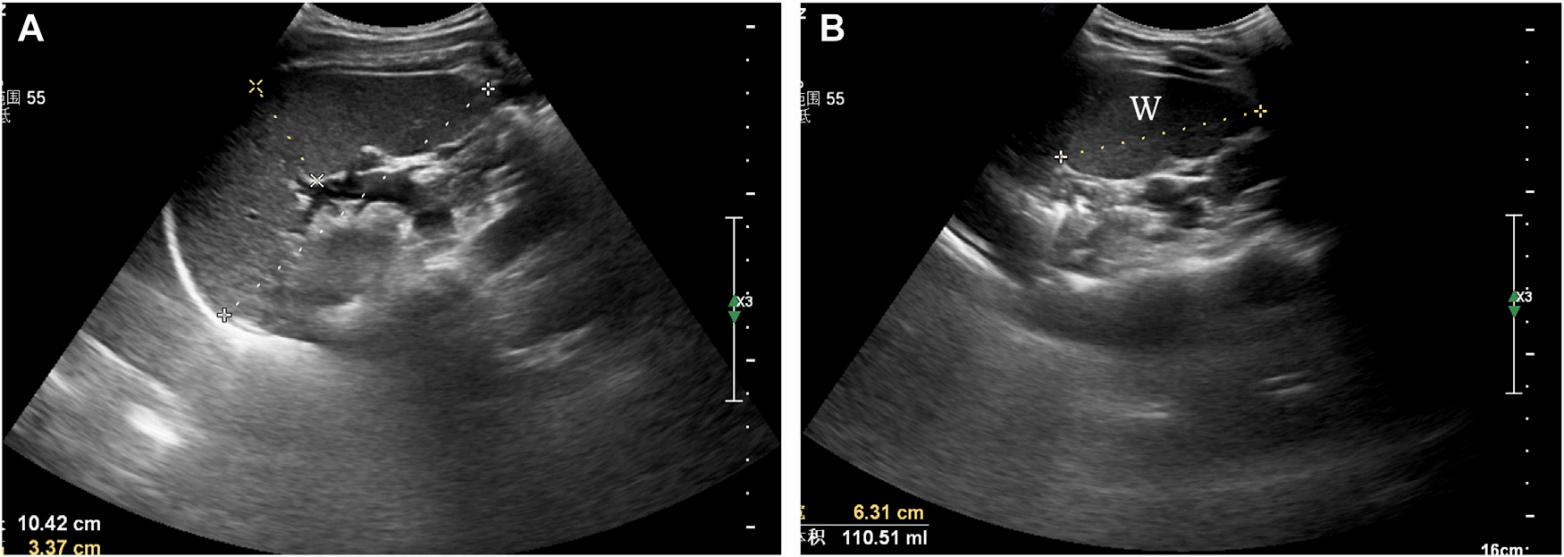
Ultrasonographic assessment of spleen volume. (A) maximum spleen length (L) and thickness (T), (B) maximum spleen width (W).
